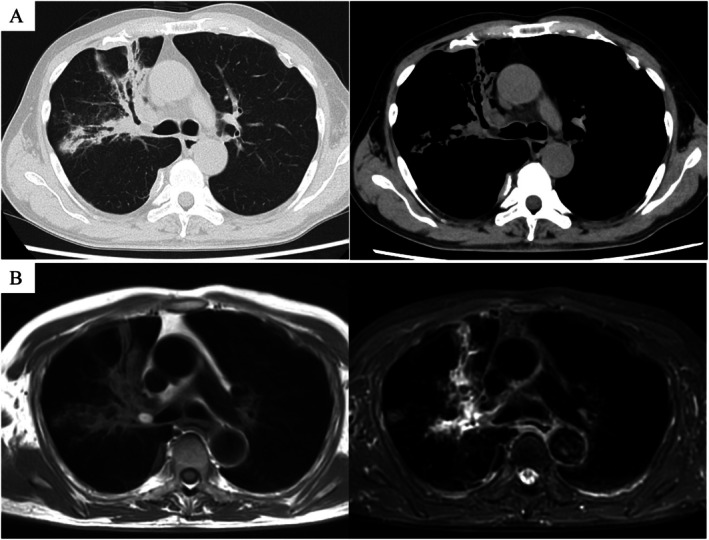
Imaging obtained before bronchoscopy. (A) The CT demonstrated a 12‐mm endobronchial tumour with low attenuation in the right upper lobe bronchus. Bronchiectasis and some infiltrative shadows, suggestive of obstructive pneumonia, were observed. (B) The tumour in the right upper lobe bronchus appeared hyperintense on T1‐weighted magnetic resonance imaging (MRI) and underwent signal suppression on fat‐suppressed T1‐weighted images. The lesion appeared hypointense on STIR images. The radiologist interpreted these findings as suggestive of a fat‐containing tumour.
